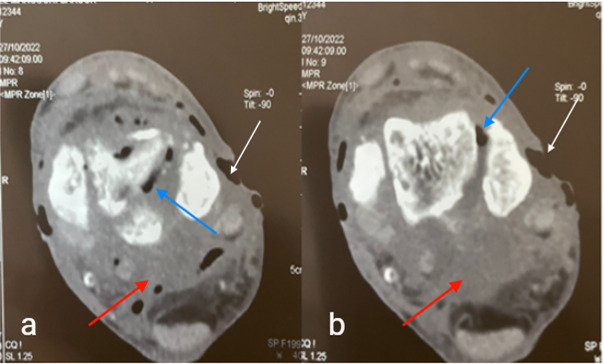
Axial ankle CT (a and b) scan showing diffuse infiltration of the soft tissues (red arrows) with significant gas extending from subcutaneous soft tissues and penetrating between bone structures (blue arrows). Note loss of cutaneous substance (white arrows).
